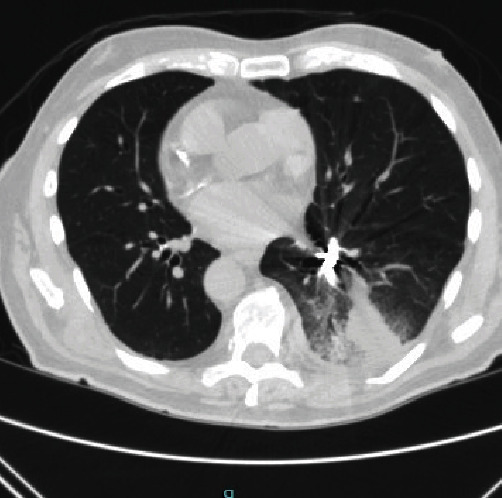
CT scan chest axial section (lung window) with a metallic object in RLL.
